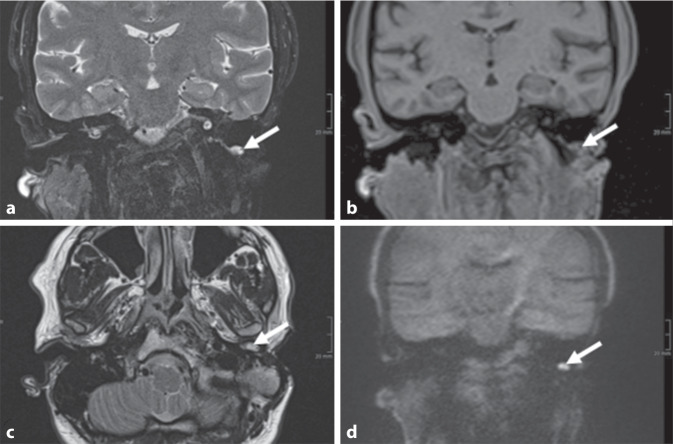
Initiale MRT unter Verwendung des Cholesteatom-Protokolls. Hier zeigt sich eine T2-hyperintense Läsion links (a koronales T2w-Bild mit Fettsättigung, c axiales T2w) dorsal des Kondylenfortsatzes, am Boden der Mittelohrhöhle. Die Läsion zeigte keine Signalverstärkung im T1w-Bild (b). In der Non-EPI-HASTE-DWI wurde eine deutliche Diffusionsrestriktion nachgewiesen (d). Daher wurde die Läsion als Cholesteatom eingeschätzt
